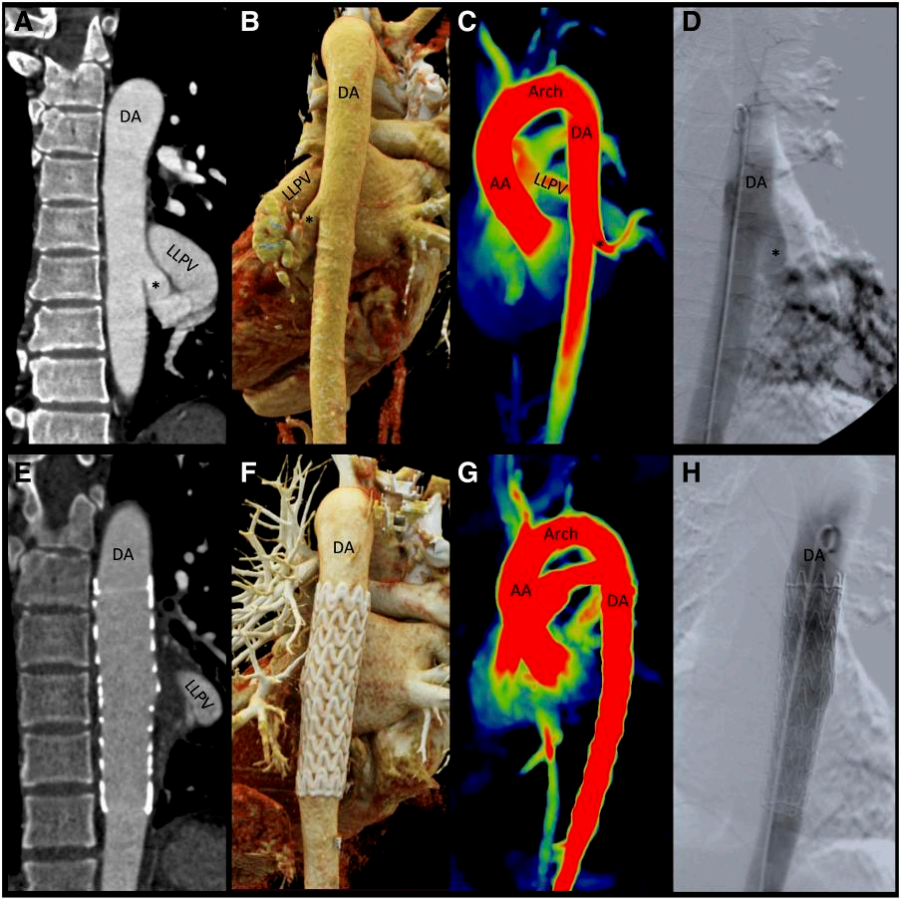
A 28-year-old man presented with recurrent haemoptysis. Chest computed tomography revealed the presence of a congenital descending aorta to pulmonary vein fistula (cDAPVF, asterisk, *Panels A* and *B*) with an aberrant vessel originating from the descending aorta and draining into a dilated left lower pulmonary vein (LLPV), diffuse alveolar haemorrhage characterized by ground glass opacities and consolidation, yet no parenchymal abnormalities to suggest sequestration (see Supplementary material online, *Video S1*). Echocardiography showed normal biventricular size and indices of systolic function. 4D flow magnetic resonance imaging highlighted the presence of a significant left-to-left shunt with an anterograde flow through the fistula of 35 mL (Qp/Qs = 0.6; *Panel C*; Supplementary material online, *Video S2*). The first-ever reported thoracic endovascular aortic repair (TEVAR) with coverage of the cDAPVF was successful (*Panels D* and *E*) in achieving swift resolution of vascular congestion (see Supplementary material online, *Video S1*) and normalization of haemodynamics (Qp/Qs = 1; *Panels F–H*; Supplementary material online, *Video S3*), with no recurrent haemoptysis at 1 year. Congenital descending aorta to pulmonary vein fistula is a rare vascular defect where aortic blood is shunted into the pulmonary venous circulation. Typically presenting in infancy with heart failure symptoms and haemoptysis, cDAPVF may remain asymptomatic until adulthood. Progressive shunting can lead to dilatation of pulmonary veins, left atrium, and pulmonary artery. Haemoptysis may occur due to rupture of small pulmonary veins. The case emphasizes the need for distinguishing cDAPVF from other vascular abnormalities, including pulmonary sequestration, scimitar syndrome, pulmonary varices, and arteriovenous malformations, as clinical implications and treatment can be profoundly different. It also highlights the role of multimodal imaging in supplying detailed anatomical and haemodynamic information for correct diagnosis and effective therapeutic guidance and introduces TEVAR as a viable treatment option for cDAPVF.
